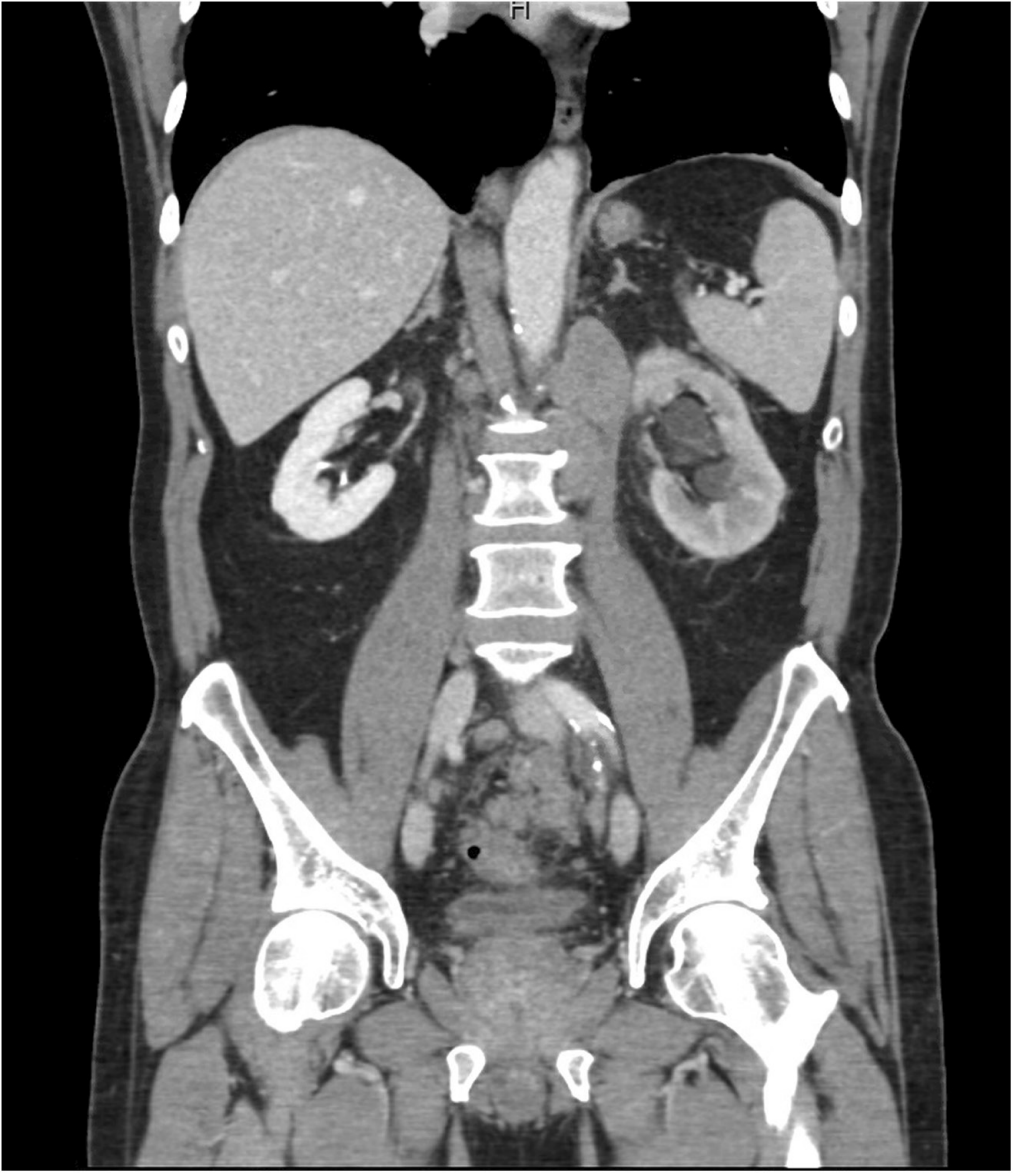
CT abdomen/pelvis with contrast (coronal view) showing obstructive retroperitoneal lymph node causing left sided hydronephrosis and hydroureter along with enlarged prostate.
